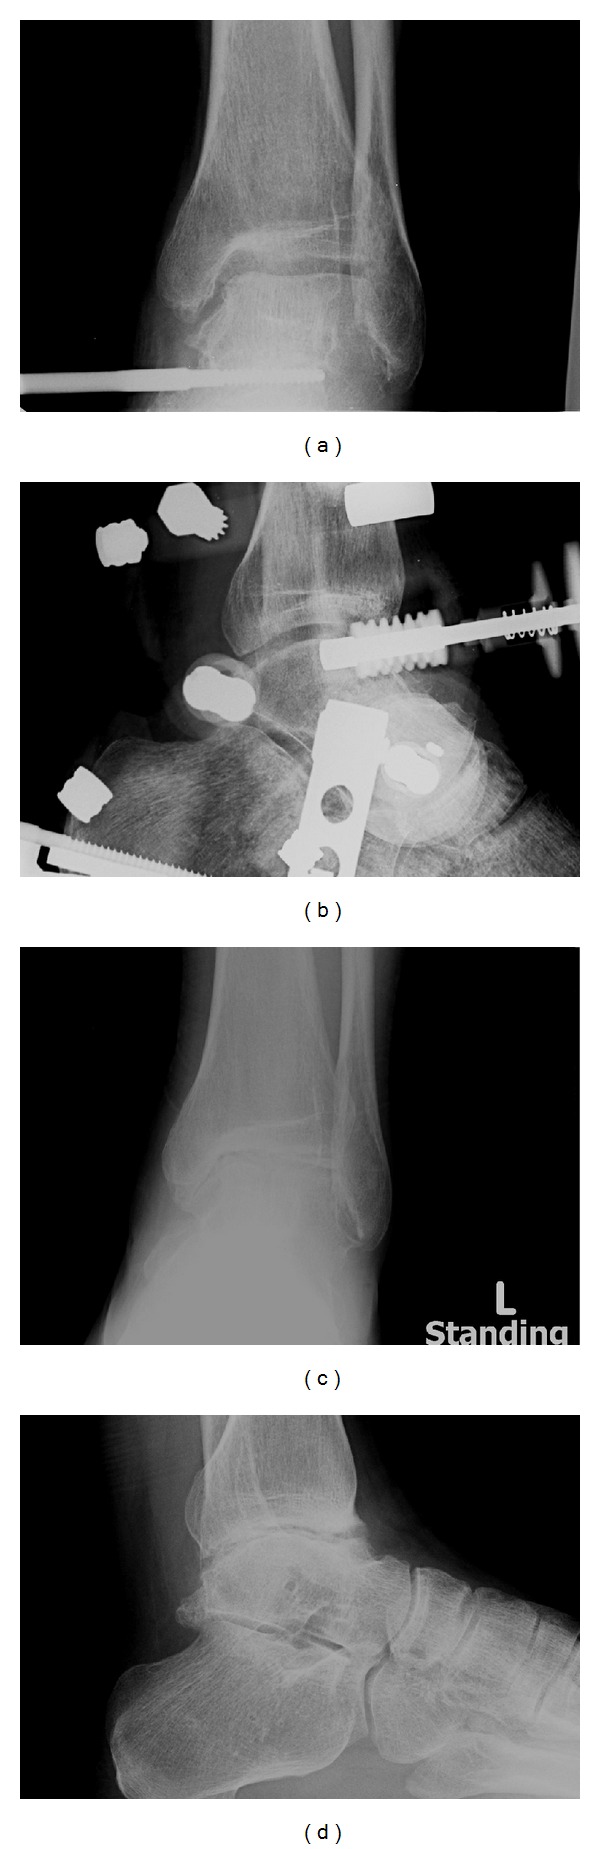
Anterolateral (a) and lateral (b) radiographs of the ankle with distraction arthroplasty showed creation of a space between the articular surfaces. (c, d) The ankle joint space remained diminished after removal of the external fixator.
